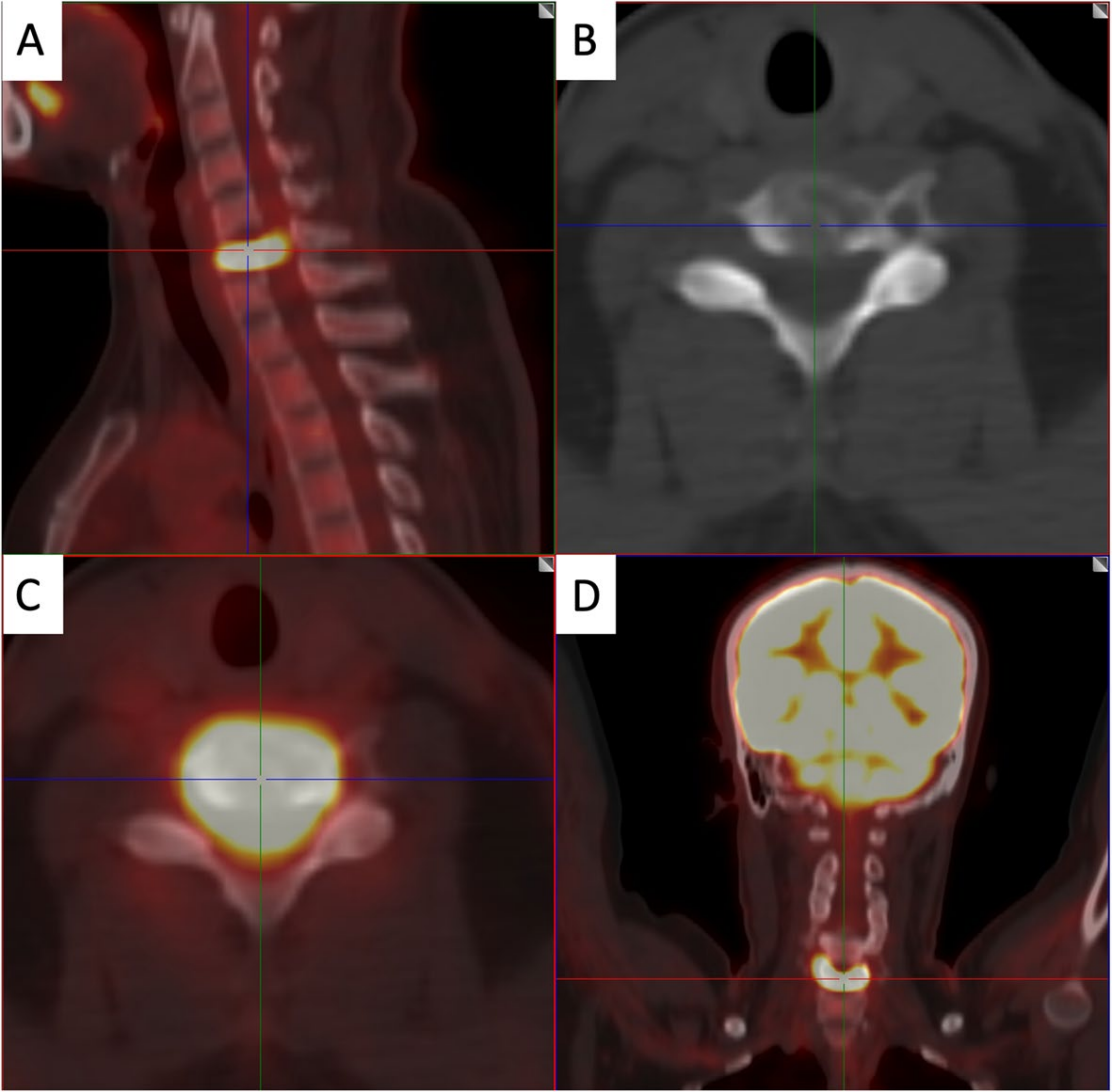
The positron emission tomography-computed tomography (PET-CT). A sagittal cut; B and C axial cuts; D coronal cut
